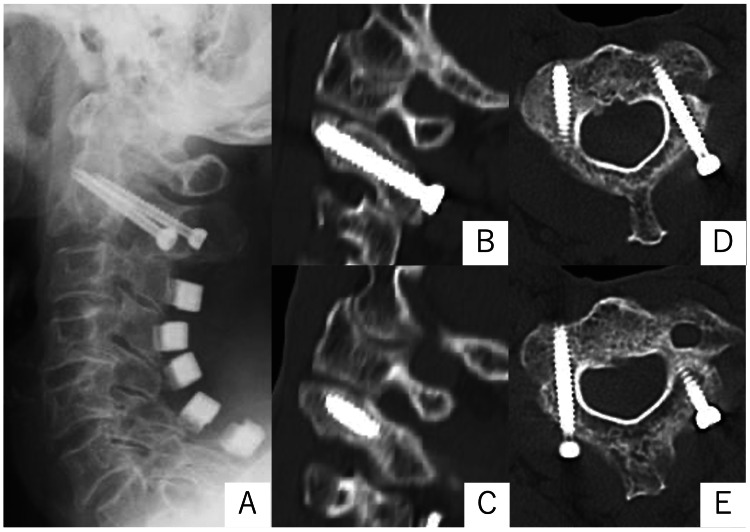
Radiographic images after surgery. X-ray obtained immediately after surgery shows that the screws are in place without any problems. (A) CT images four months later already confirmed acceptable fracture healing. B, C: sagittal; B: right; C: left; D, E: axial.
